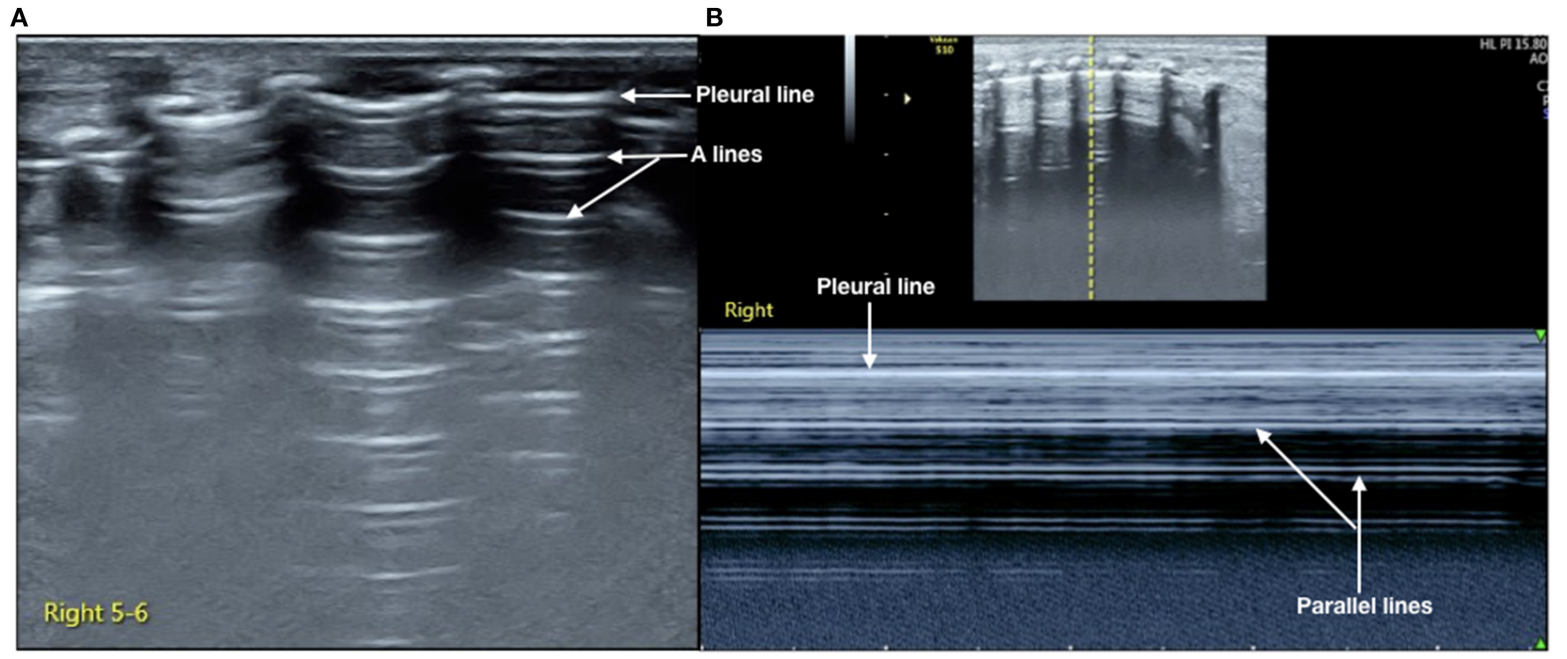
Lung ultrasonography (LUS) of the right chest. LUS examination on anterior, subaxillary, and posterior chest away from the spine. (A) B-mode ultrasound, we can see that the pleural line and A-line clearly existed but no B-line was found. (B) M-mode ultrasound; it presented as a stratosphere sign. It was confirmed as the severe PTX according to the *International Expert Consensus and Recommendations for Neonatal Pneumothorax Ultrasound Diagnosis* (3).
